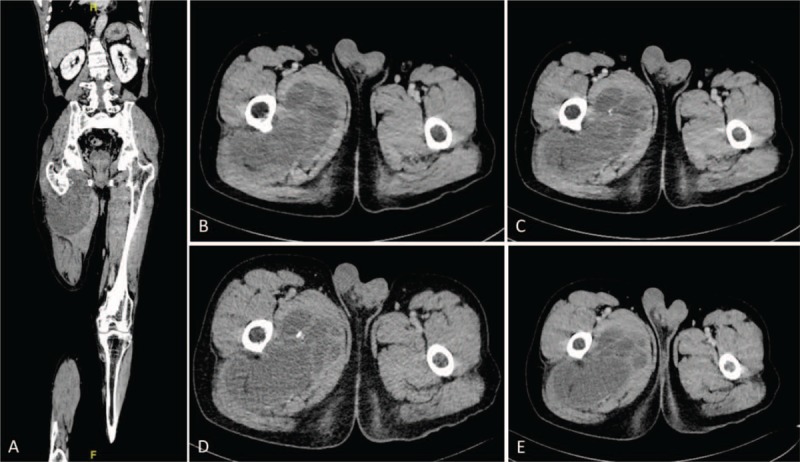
(A–E) Preoperative coronal and transverse CT scan revealed multilocular cystic-solid mass in the right thigh root, with high suspicion of malignancy.
